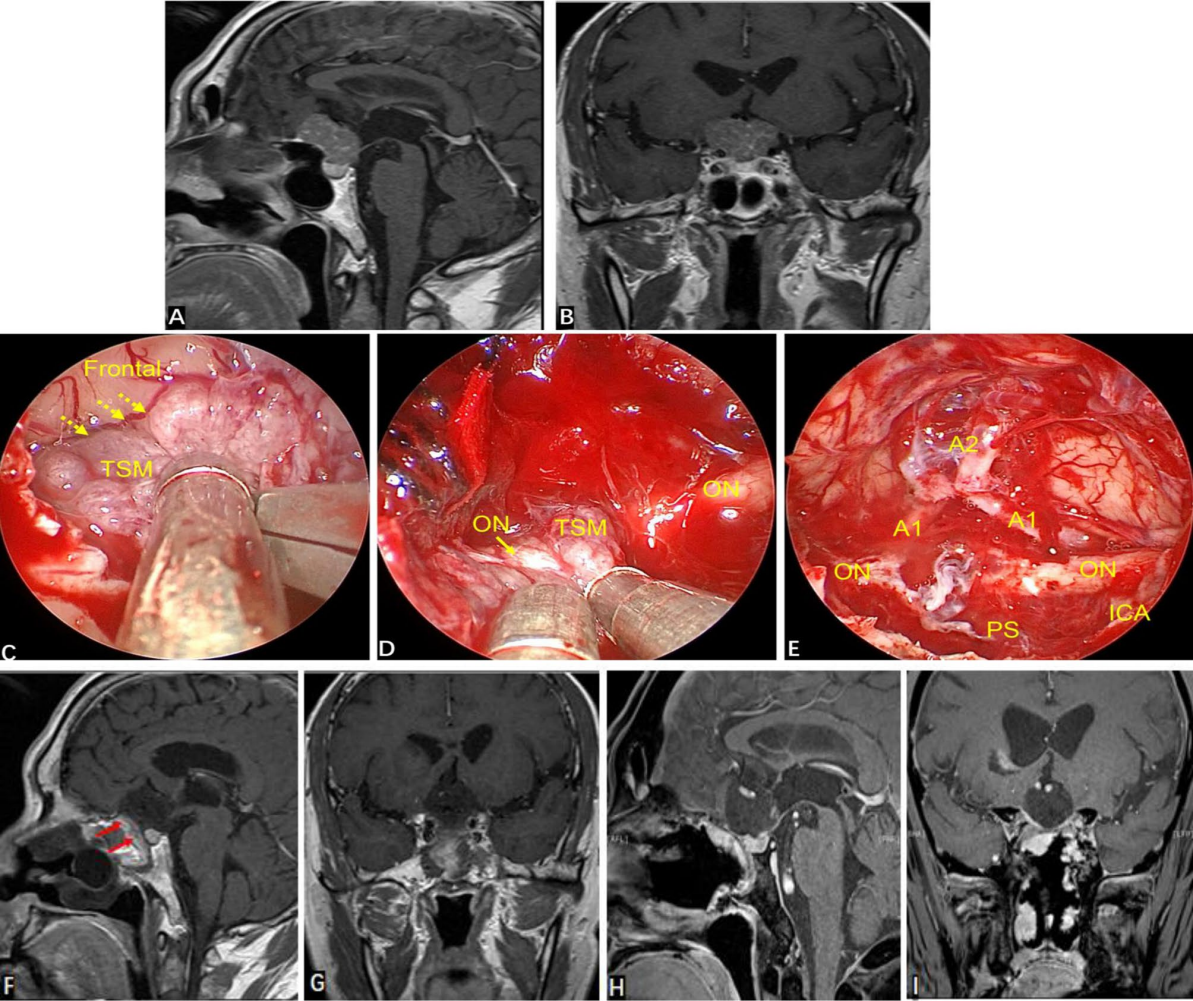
(A,B) Preoperative contrast-enhanced MRI of the sellar region indicated that the tumor was closely related to the ACA and ICA. (C) The tumor had no arachnoid plane, and adhered widely to the frontal lobe (yellow dotted-line arrow). (D) The right optic nerve had been invaded, and adhesion with the tumor was very severe. (E) After total resection of the tumor, invasion of the right optic nerve was revealed. (F,G) Contrast-enhanced MRI of the sellar region at POD3 suggested total resection of the tumor, and the VNF healed well (red solid-line arrow). (H,I) Six months after surgery, contrast-enhanced MRI of the sellar region showed no sign of residual tumor or recurrence.
